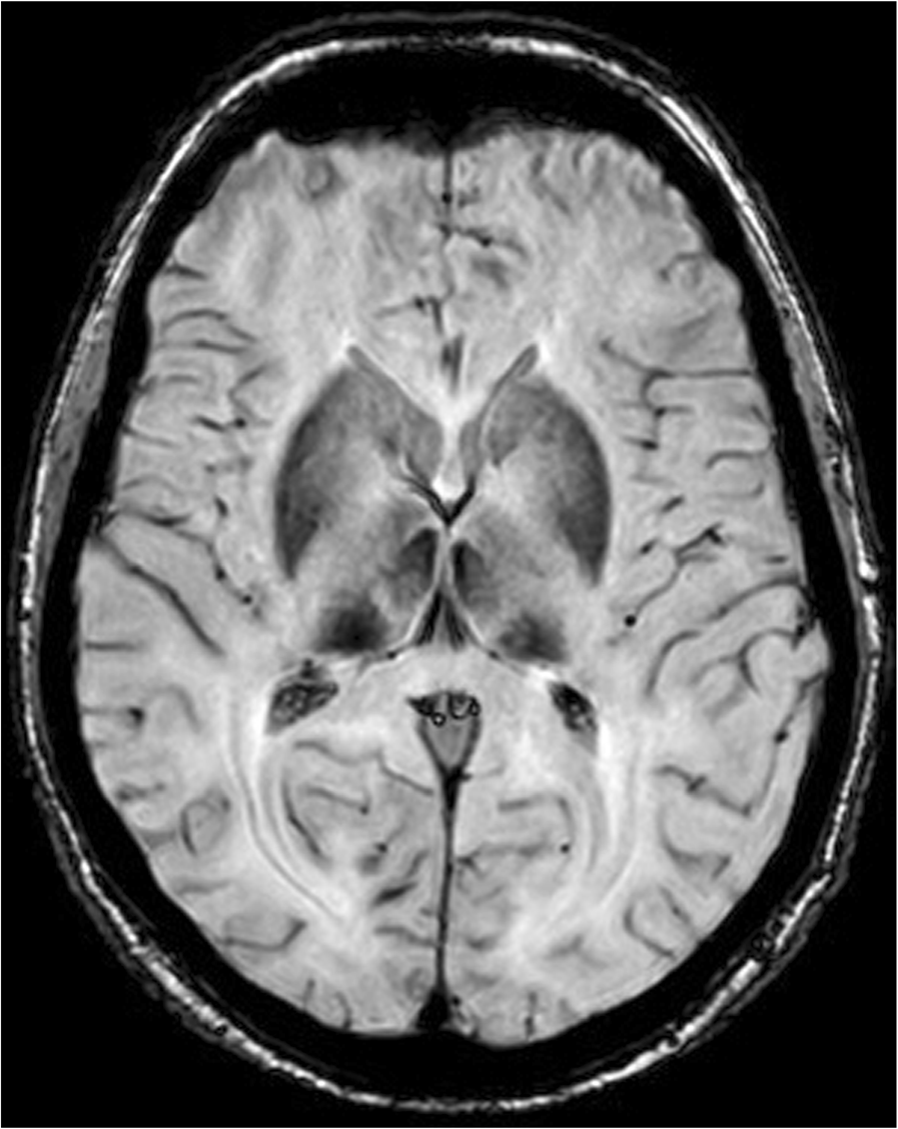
MRI of the brain. Susceptibility weighted imaging. Hypo-intensive changes in the basal ganglia and thalamus caused by deposits of iron
